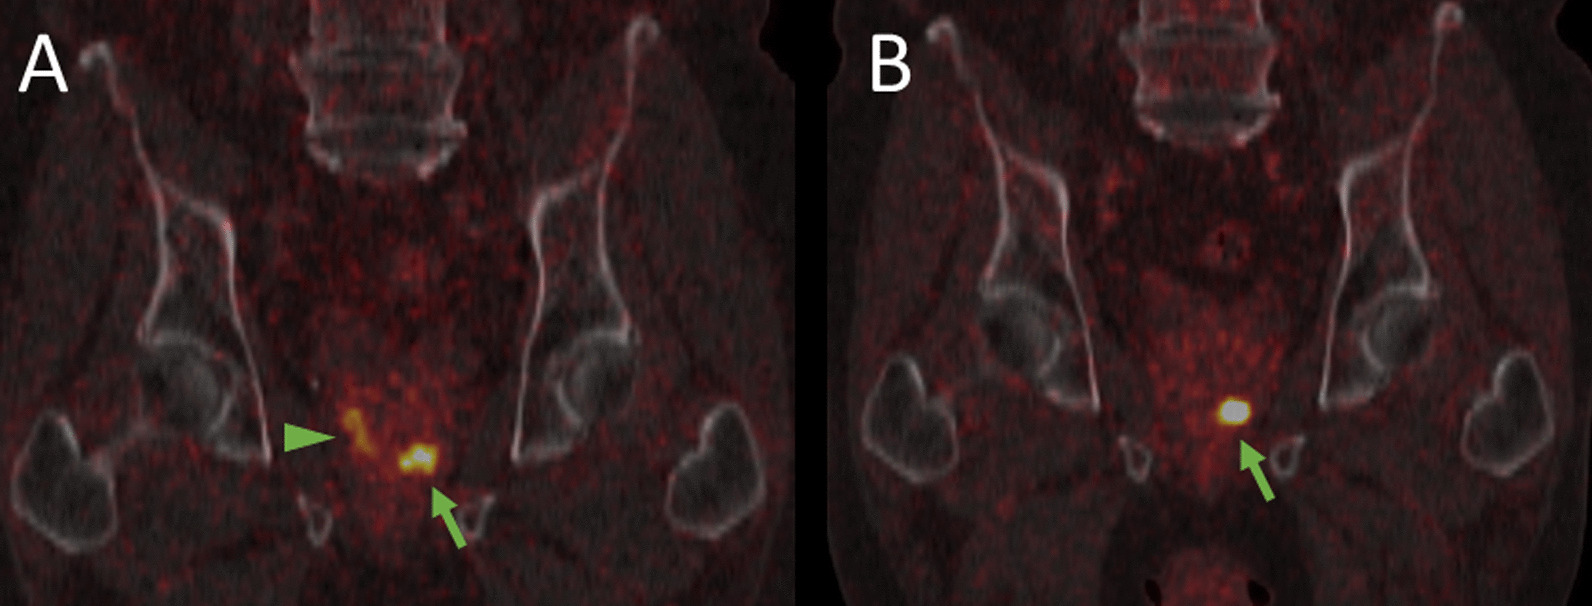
Coronal fused [^68^Ga]-Ga-PSMA-11 (A) and [^18^F]-F-PSMA-1007 PET/CT (B). Concordant focal uptake in the primary tumor in the left apical lobe (green arrow). The longitudinal uptake in the peripheral lobe in the [^68^Ga]-Ga-PSMA-11 PET/CT (arrowhead) finds no counterpart in [^18^F]-F-PSMA-1007 PET/CT
